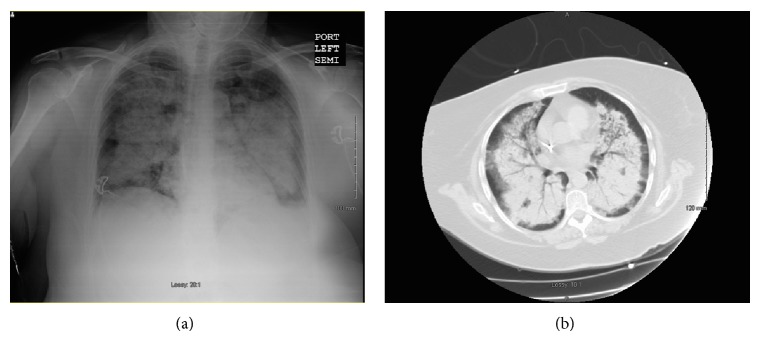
(a) Chest X-ray showing bilateral pulmonary infiltrates in an airspace pattern. (b) Computed tomography of the chest without contrast showing airspace opacities bilaterally sparing the periphery.
